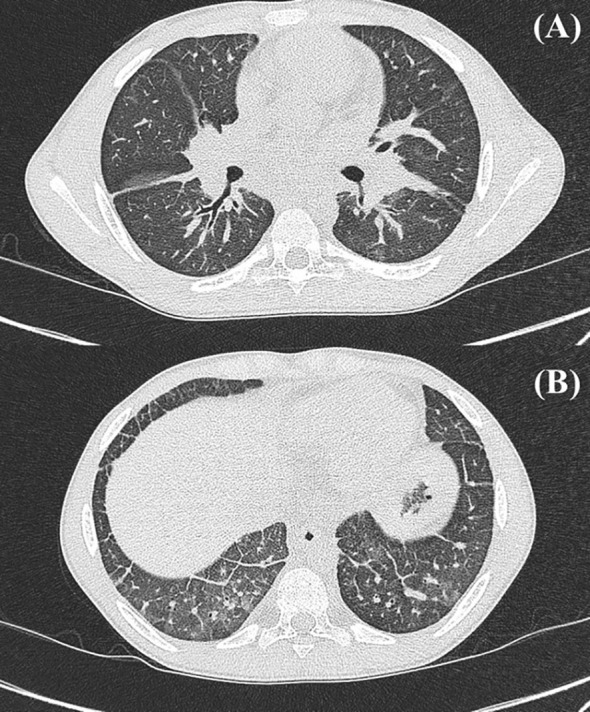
Case 1 chest computed tomography scan prior to rituximab–cyclophosphamide induction. sJIA-LD characterized by the following: (A) Interlobular septal and peribronchial thickening. (B) Bronchiectasis and peribronchial thickening in the bilateral lower lobes. Thickening most prominent in dependent portions of the lungs. sJIA-LD, systemic juvenile idiopathic arthritis-associated lung disease.
